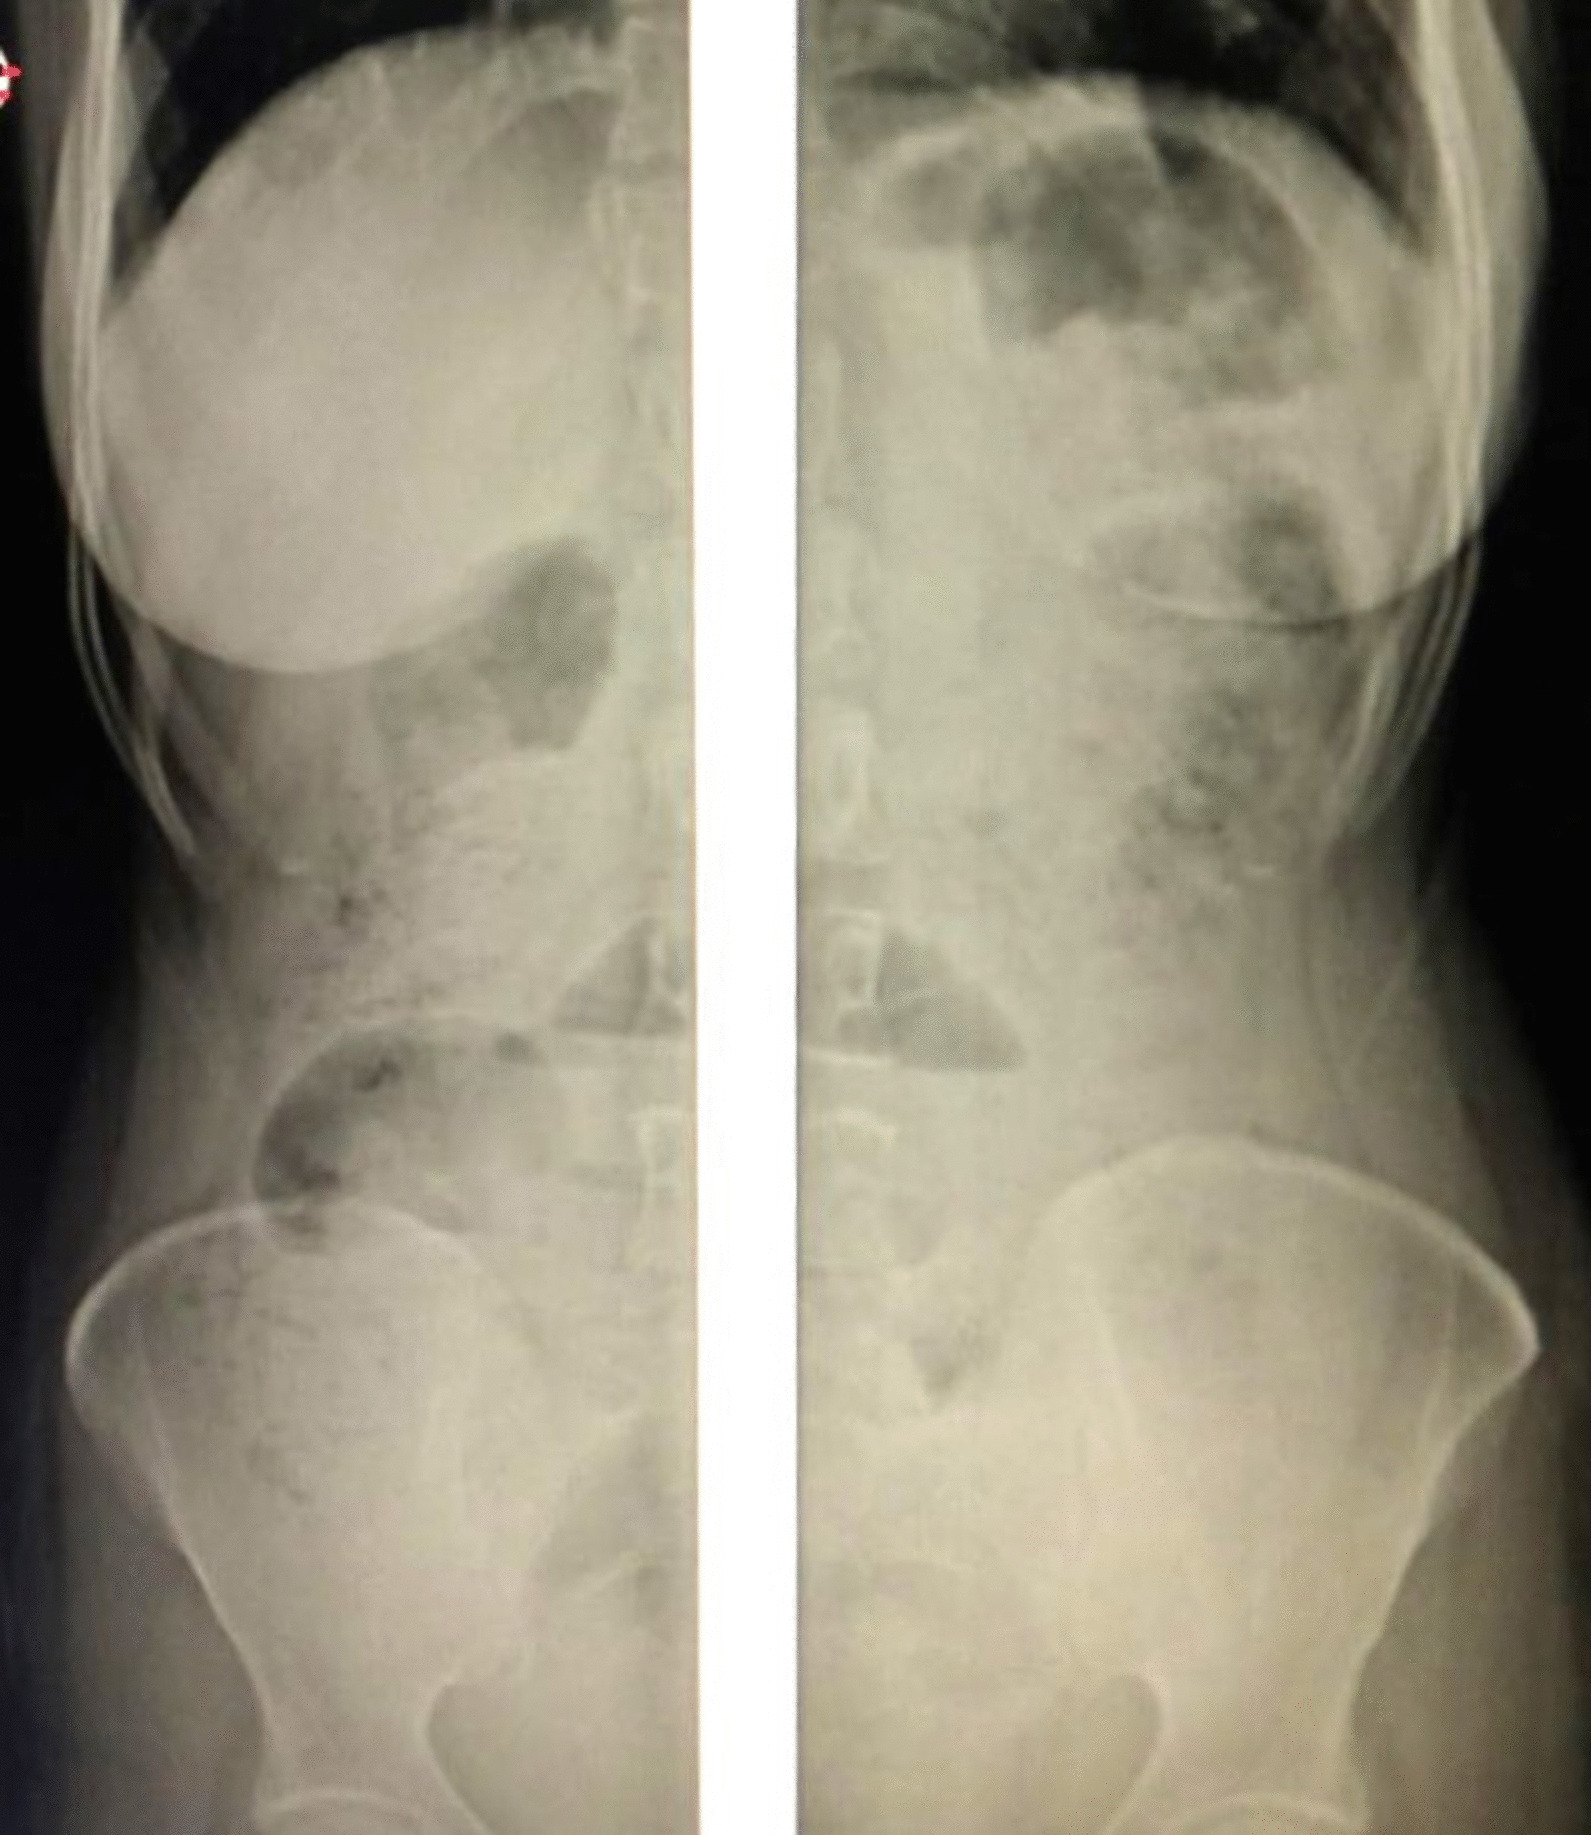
preoperative X-ray showing dilated small bowel loops with air-fluid level
